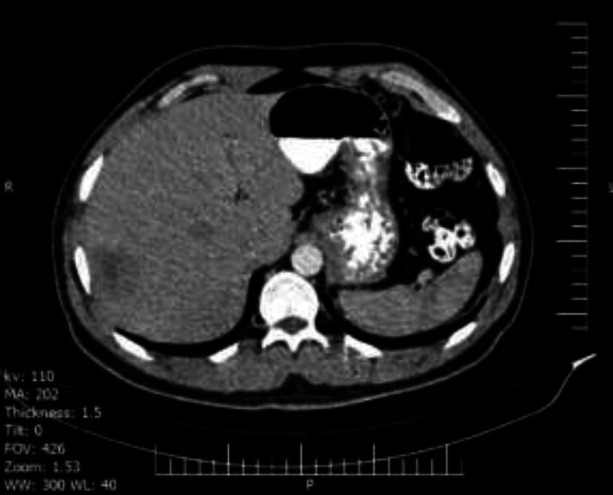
Abdominal CT revealed multiple bilateral hepatic mass lesions with the largest size being (41*32 mm) at segment 7, and 28*2 (3)7 mm at segment 3
